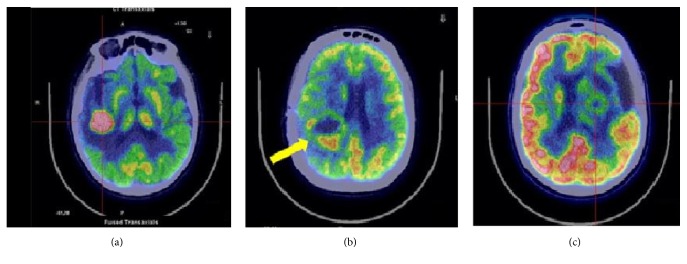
Axial ^18^F-FDG brain imaging. PCNSL (a), glioblastoma multiforme (b), and abscess (c).
